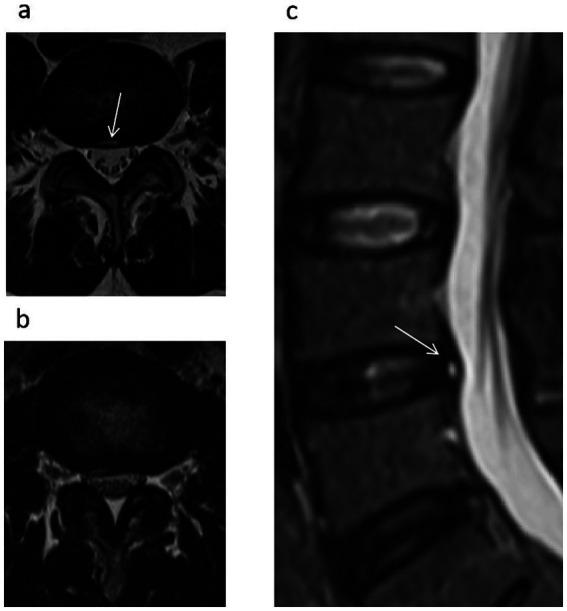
SedSign: (a) Negative; (b) Positive. T2-weighted axial (a) and sagittal (c) images demonstrating an HIZ within the central posterior annulus at the L4/5 level as indicated by the white arrow.
